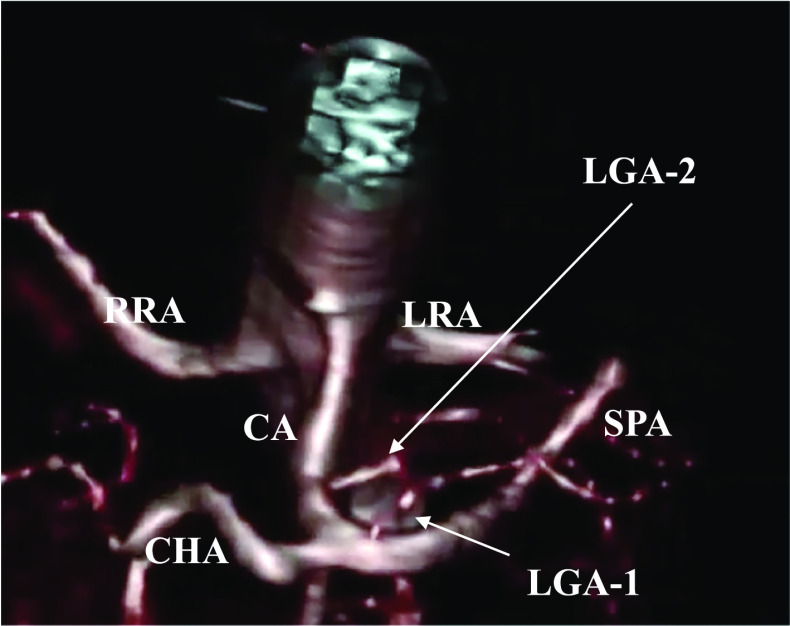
Caudal view of the three-dimensional-computed tomography angiogram demonstrating the LGA-1 branching from the root of the splenic artery and the LGA-2 arising from the celiac artery. *CA* celiac artery, *CHA* common hepatic artery, *SPA* splenic artery, *RRA* right renal artery, *LRA* left renal artery, *LGA* left gastric artery
